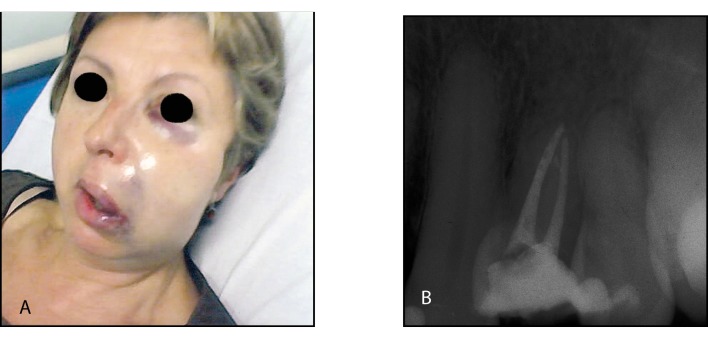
Case report nº2. (A) Frontal view of the patient following sodium hypochlorite extrusion in the upper left first premolar. (B) Periapical radiography of the affected premolar following root canal treatment.
